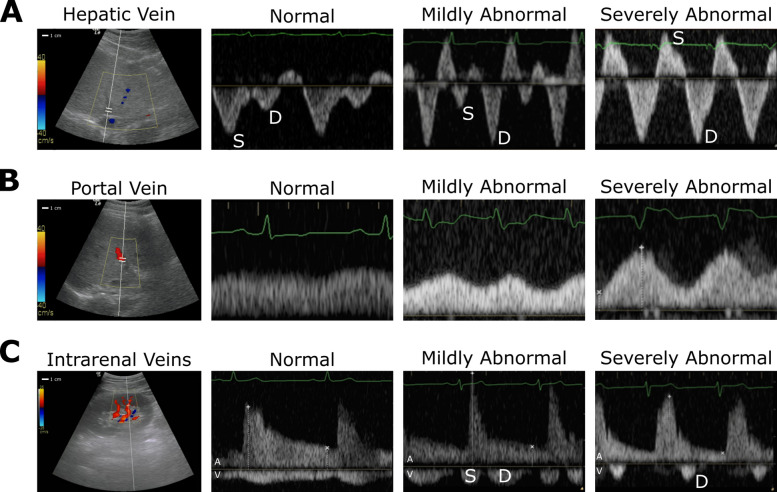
Representative hepatic, portal, and intrarenal vein imaging sites and pulse-wave Doppler signals obtained when performing a Venous Excess Ultrasound Score (VExUS). A Hepatic vein Doppler waveforms demonstrating normal (systolic [S] wave greater than diastolic [D] wave), mildly abnormal (D wave greater than S wave), and severely abnormal (S wave reversal) patterns. B Portal vein Doppler waveforms demonstrating normal (< 30% pulsatility index), mildly abnormal (30%–49% pulsatility index), and severely abnormal (≥ 50% pulsatility index) patterns; pulsatility index = (maximum velocity – minimum velocity)/maximum velocity. C Intrarenal vein Doppler arterial (A) and venous (V) waveforms demonstrating normal (monophasic flow), mildly abnormal (discontinuous, biphasic flow with systolic [S] and diastolic [D] phases), and severely abnormal (discontinuous, monophasic flow with diastolic [D] phase only). Reused from Dinh [82], with permission from POCUS 101
